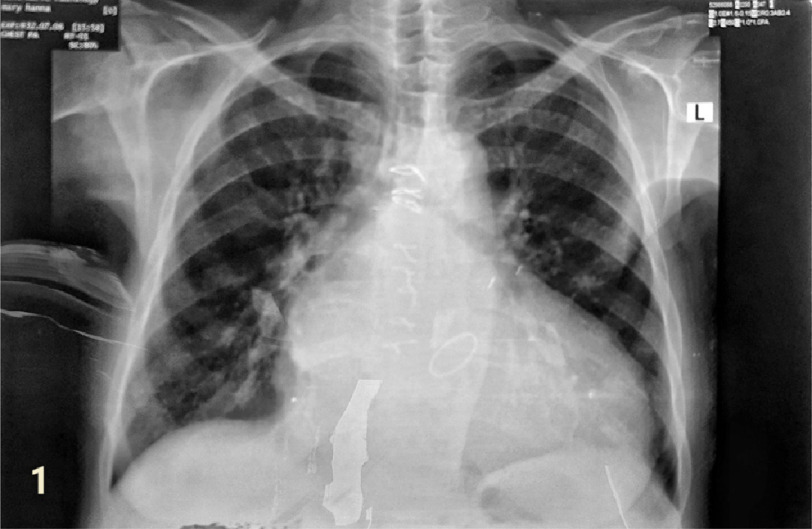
Chest x-ray showing enlarged LA, biventricular enlargement, and pulmonary venous congestion.
